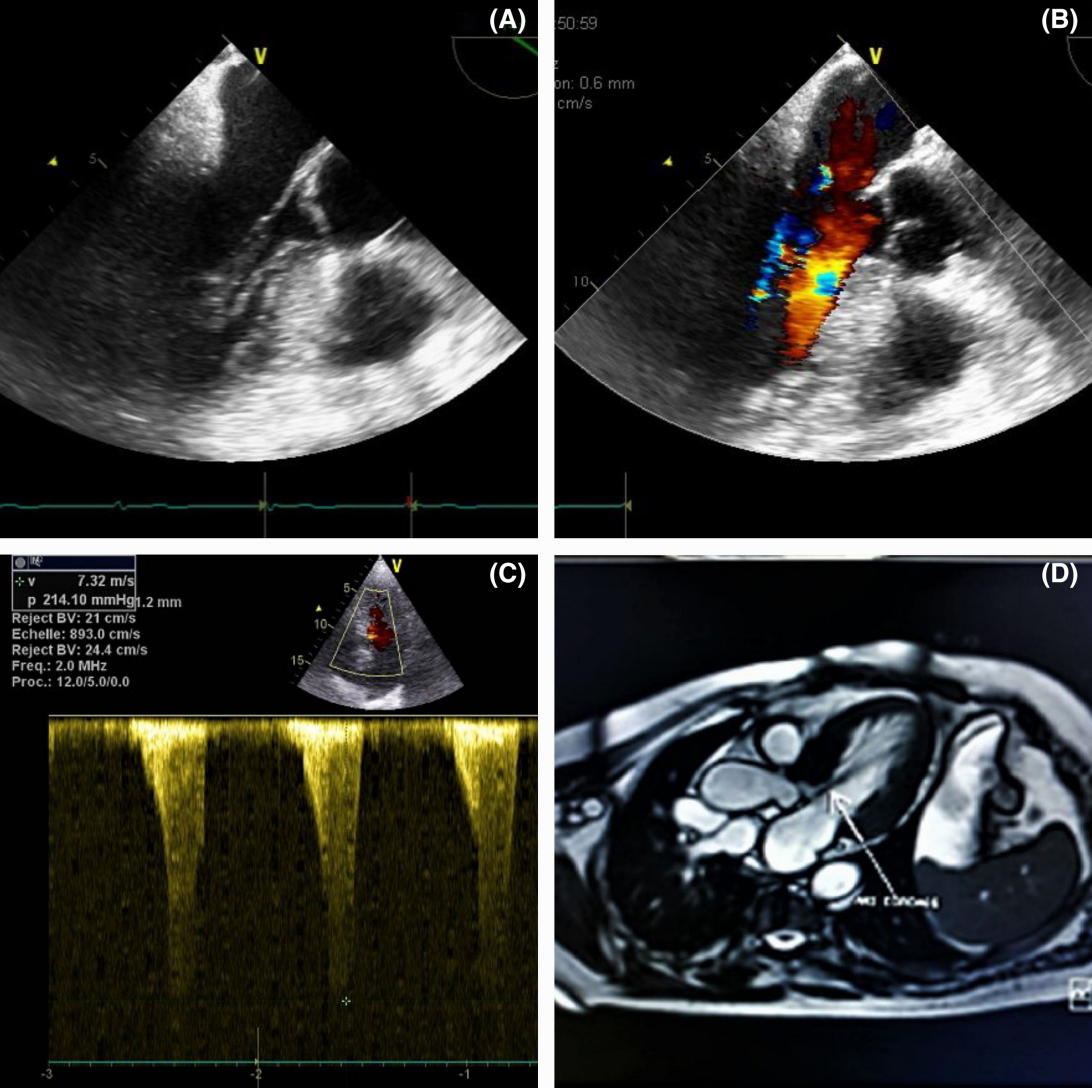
Imaging findings. (A) Apical view shows a significant LVOT narrowing. The septal wall showed increased thickness measured at 20 mm. Two subvalvular structures attached to the interventricular septum and the mitro‐aortic trigona were also visualized (blue arrows). (B) Color Doppler reveals a flow acceleration with aliasing across the LVOT (yellow arrow) with a moderate mitral regurgitation (orange arrow). (C) Continuous‐wave Doppler at the LVOT showing a peak velocity of 7.32 m/s and a maximal gradient ≈of 210 mm Hg. The waveform contour is dagger‐shaped. (D) MRI: Abnormal insertion of the chordae confirmed by MRI, obstruction which is more likely caused by septal hypertrophy than abnormal chordae.
